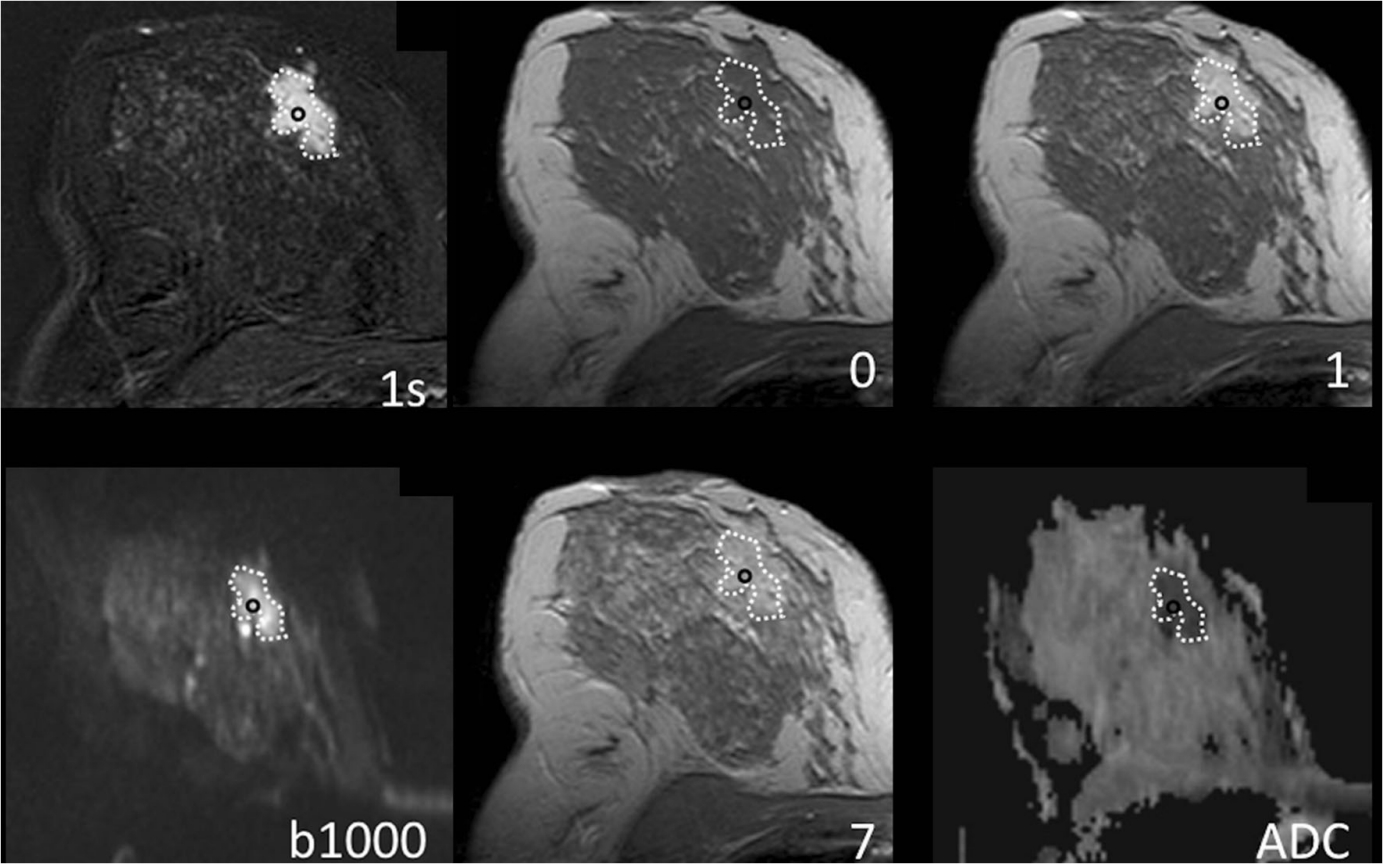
Breast MRI of a 41-year-old woman with invasive ductal cancer grade 2. Numbers denote acquisition time points of T1-weighted dynamic gradient echo images after intravenous contrast medium injection in minutes (0 = precontrast acquisition). 1s equals first subtraction. The dotted line delineates the lesion from the surrounding breast parenchyma. The lesion is depicted as an ill-defined mass lesion with fast initial-phase enhancement and wash-out during the delayed phase. The region of interest to extract the diagnostic information used in this work is highlighted by a black circle
